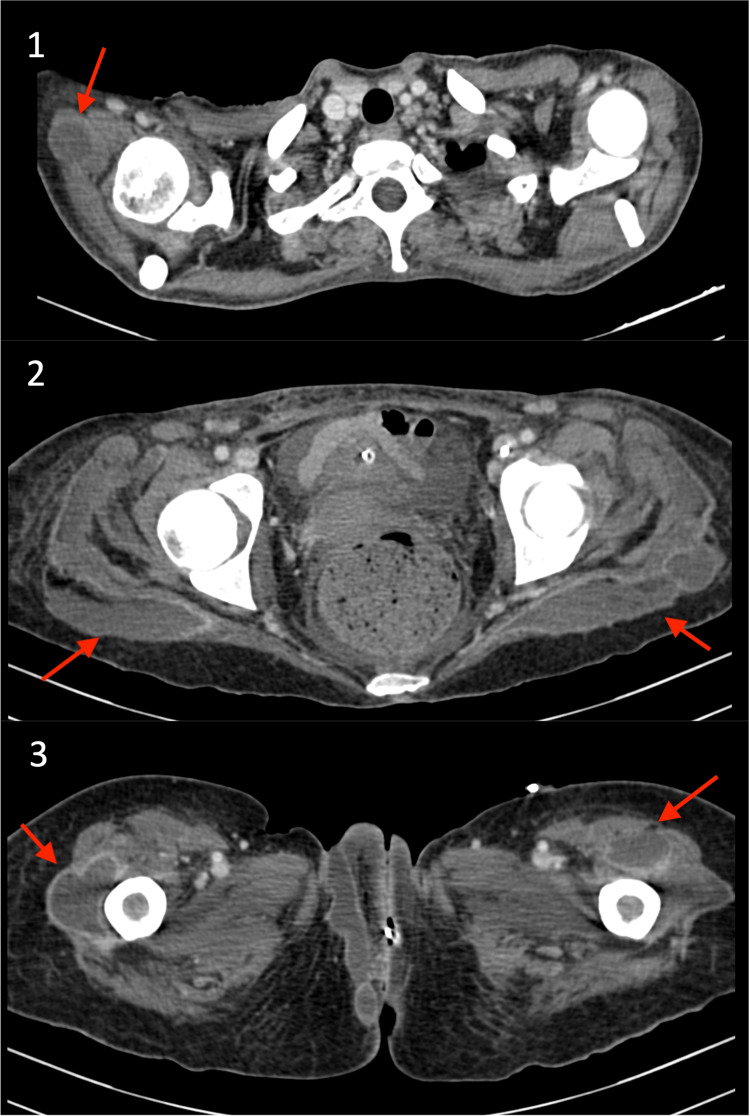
CT scan performed on the 30th day (1) Collection adjacent to the right humerus, measuring 26 × 24 mm. (2) In both gluteal regions, on the right measuring 86 × 28 mm and on the left measuring 106 × 32 mm. (3) On both femurs, on the right measuring 26 × 47 mm and on the left measuring 26 × 39 mm. The arrows show abscesses.
